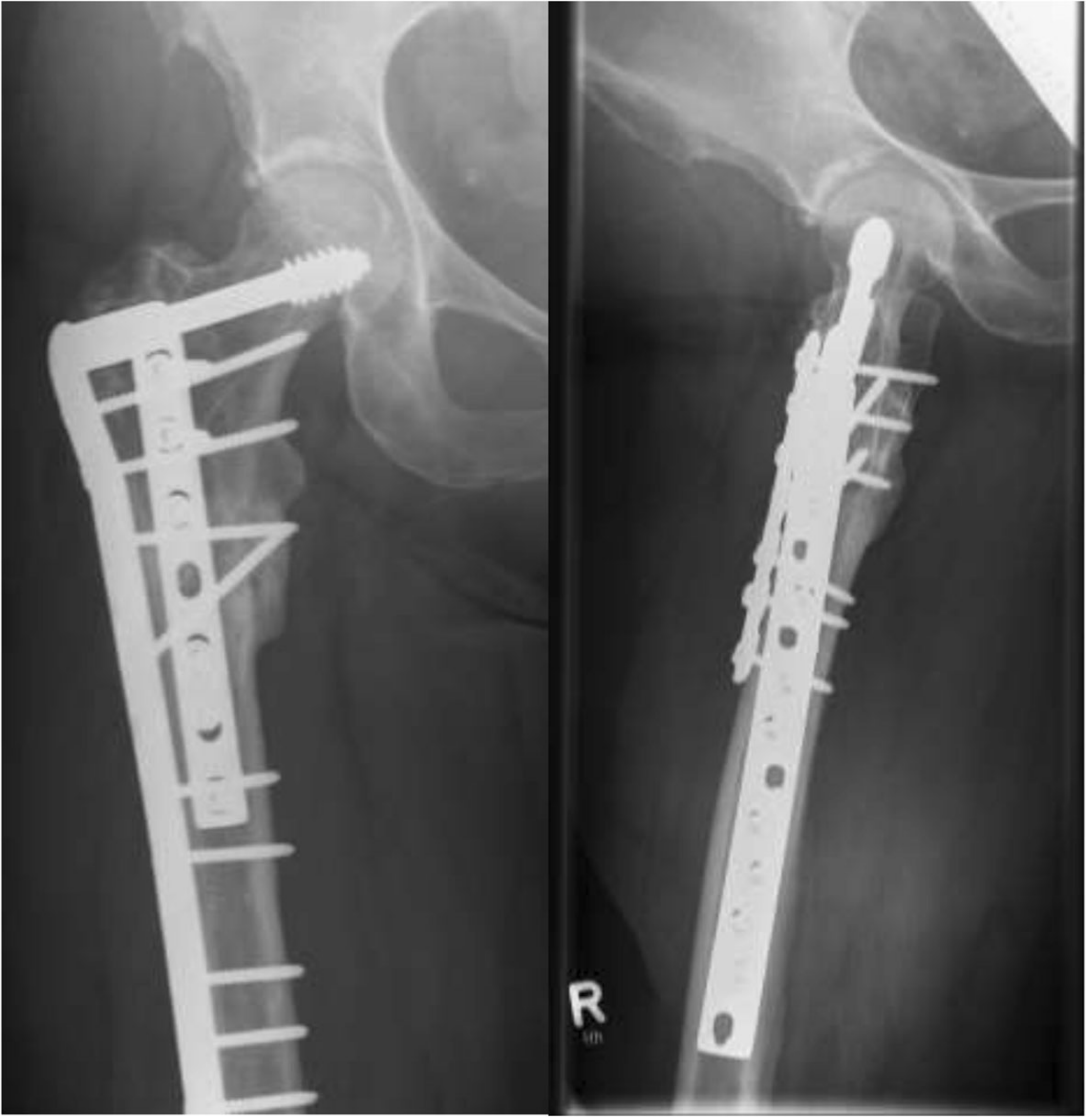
X-rays of the same patient showing a healed nonunion six months after index treatment
